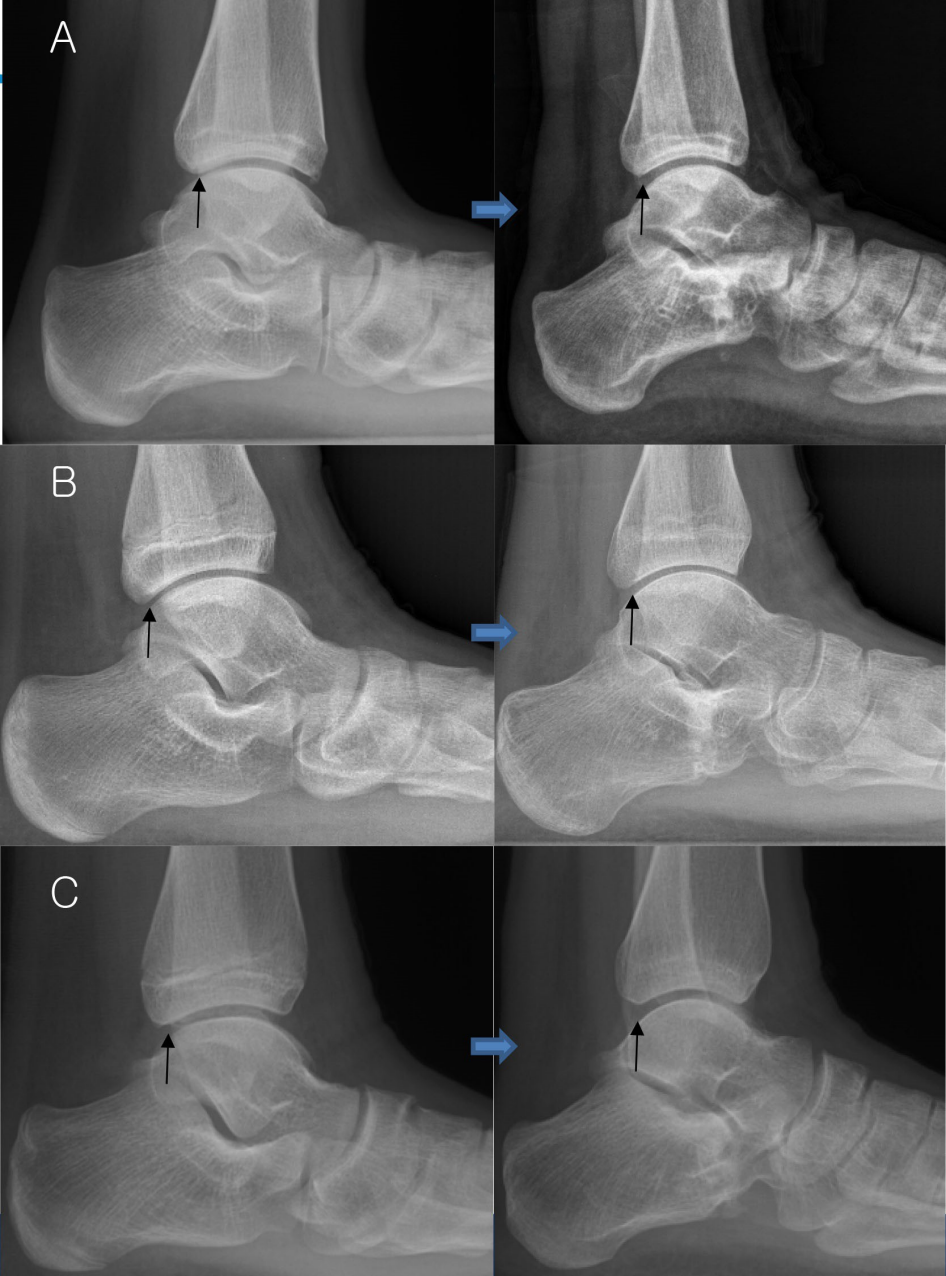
Postoperative radiographic change following calcaneal lengthening. A: A narrow posterior ankle joint interval (arrows) shows improvement at 2 years and 6 months postoperatively in a 20-year-old man. The talus has been dorsiflexed and anteriorly displaced postoperatively. B: A similar postoperative radiographic change in a 14-year-old boy at 11 months postoperatively. C: Another example in a 14-year-old girl at 1 year and 8 months postoperatively.
